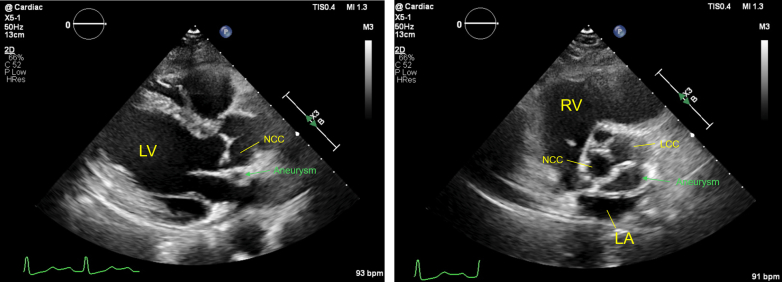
Transthoracic Echocardiography at the Outpatient Visit Transthoracic echocardiography revealed a membranous structure near the sinus of Valsalva on the side of the LCC and NCC. LA = left atria; LCC = left coronary cusp; LV = left ventricle; NCC = noncoronary cusp; RV = right ventricle.
